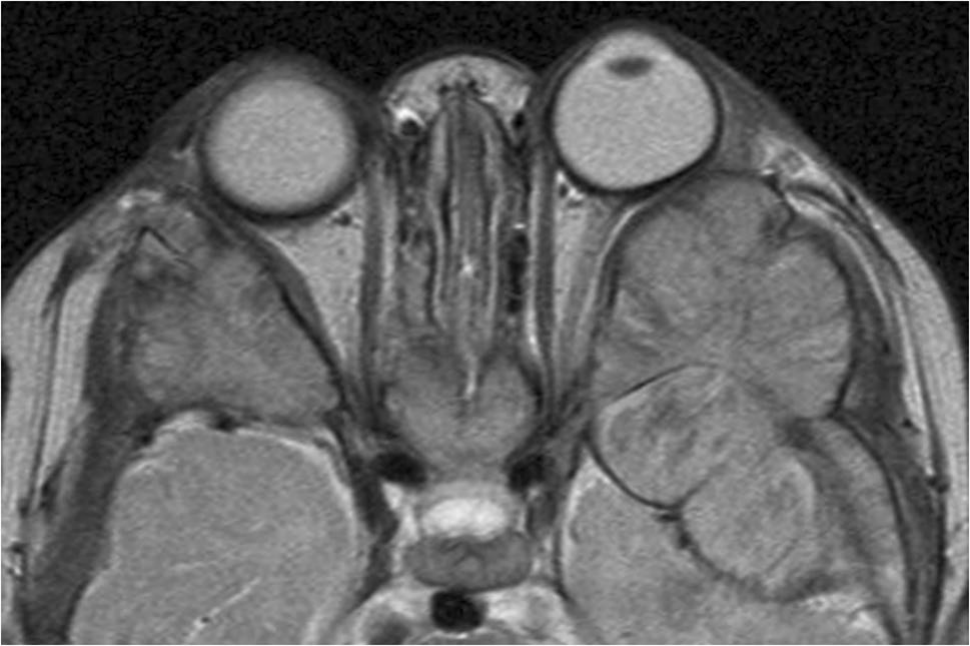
Neuroblastoma metastases. Axial T2-weighted image shows left greater than right expansile masses centered at sphenoid wings with displacement of the globe and intraorbital contents. Striated appearance of the lesions corresponds to the spiculated periosteal reaction
